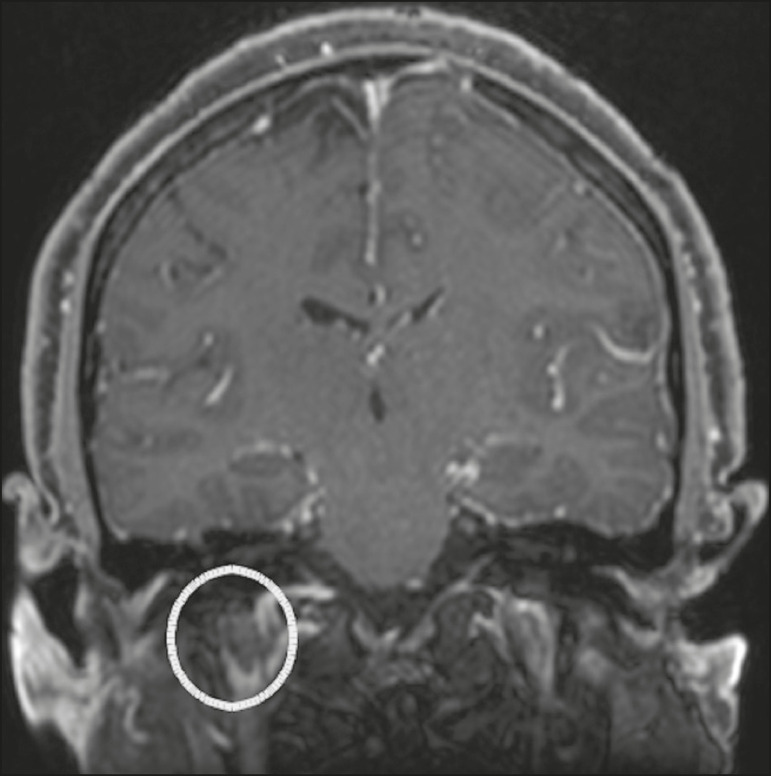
Venous thrombosis. A 36-year-old male presenting with dysphagia, dysphonia, and right-sided neck pain. Coronal contrast-enhanced T1-weighted MRI scan showing a focal thrombus within the right jugular bulb and the cranial segment of the internal jugular vein, near the jugular foramen, along the course of the ninth through twelfth cranial nerves (circle), which explained the symptoms.
